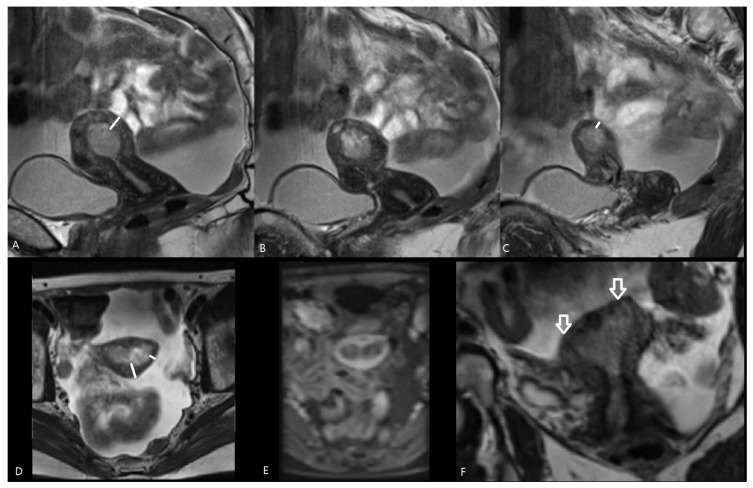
MRI of endometrial cancer. Sagittal T2-WI (A–C), axial T2-WI (D), axial T1-WI after Gadolinium administration (E) and coronal T2-WI (F). The tumor is extending intraluminal into the left and right cornu (white arrows on (F)). In the cornua, the myometrium is anatomically thinner (short white line in (C,D)) than in the uterine corpus (long white line in (A,D)), which may result in overstaging because intracavitary growth in the cornu (pT1a) may mimic tumor invasion in the outer half of the myometrium (iT1b).
